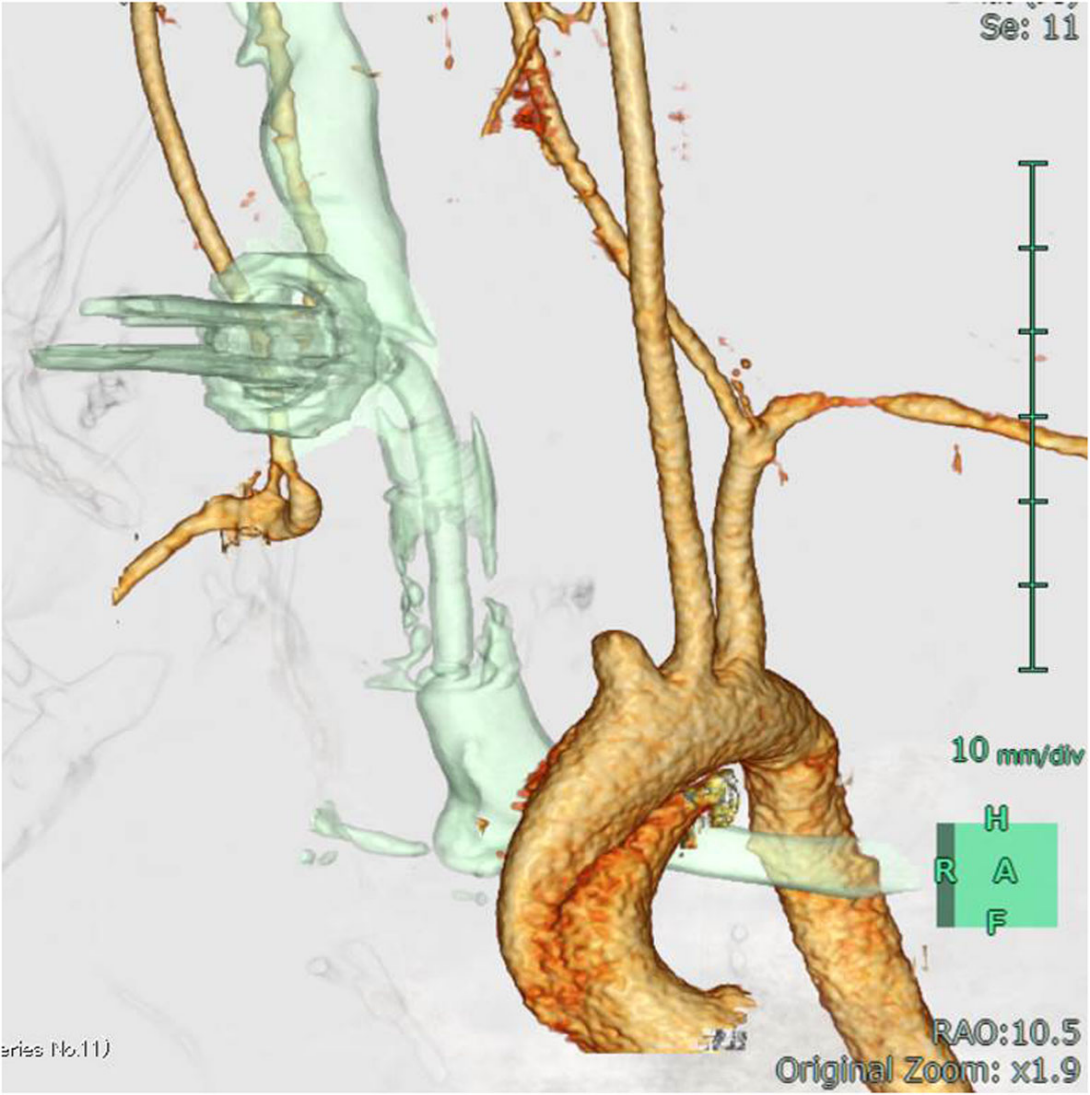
Post-operative CECT in Case 2. Post-operative CECT revealed the innominate artery, which was successfully transected (CECT: contrast-enhanced computed tomography)
